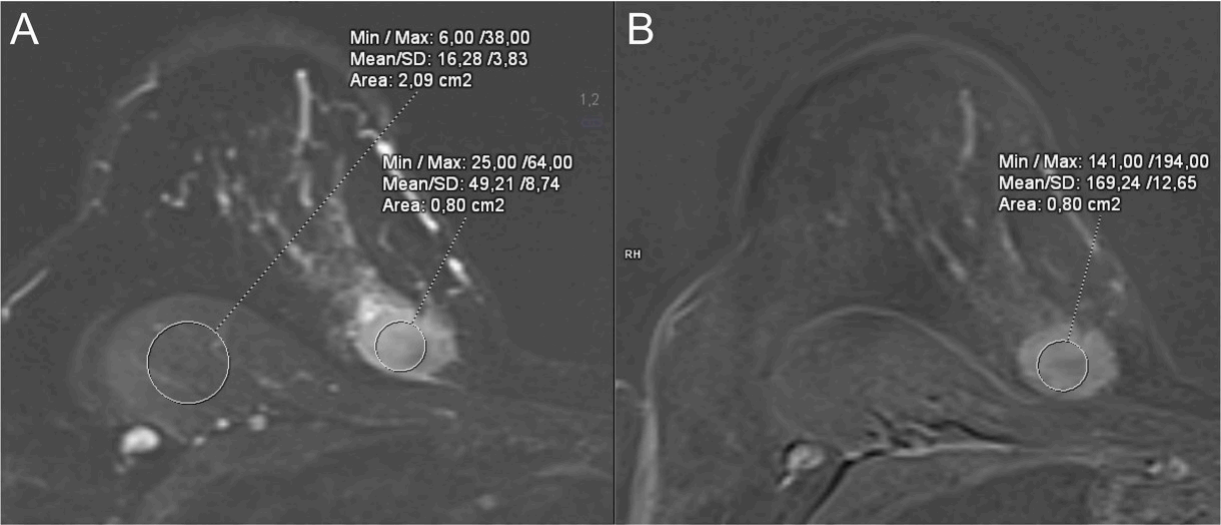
Assessment of T2w signal intensity (SI). (A) T2w SI was assessed on fat-saturated T2-weighted sequences. The ROI mask (see main task) was copied to this sequence from the contrast-enhanced T1w sequence (B). The corresponding mean SI was normalized to the mean SI of the pectoralis major muscle. In this example, this resulted in a T2w SI of 3.0 (49.2/16.3).
